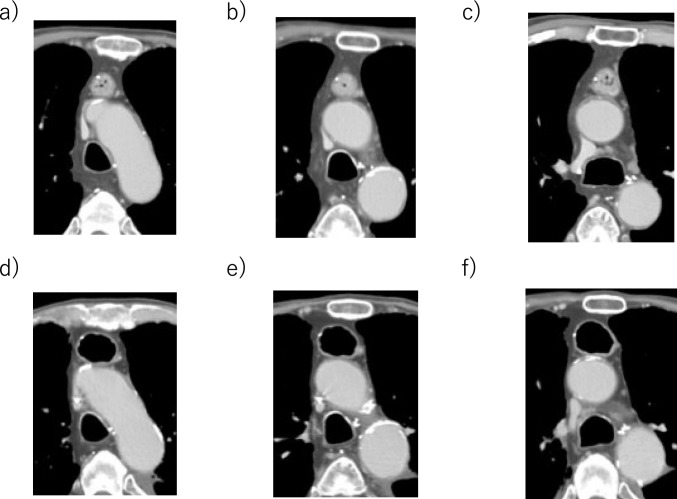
Axial thoracic computed tomography images for case 20 in Table 1. a–c Images obtained 3 months after surgery. d–f Images obtained 45 months after surgery
